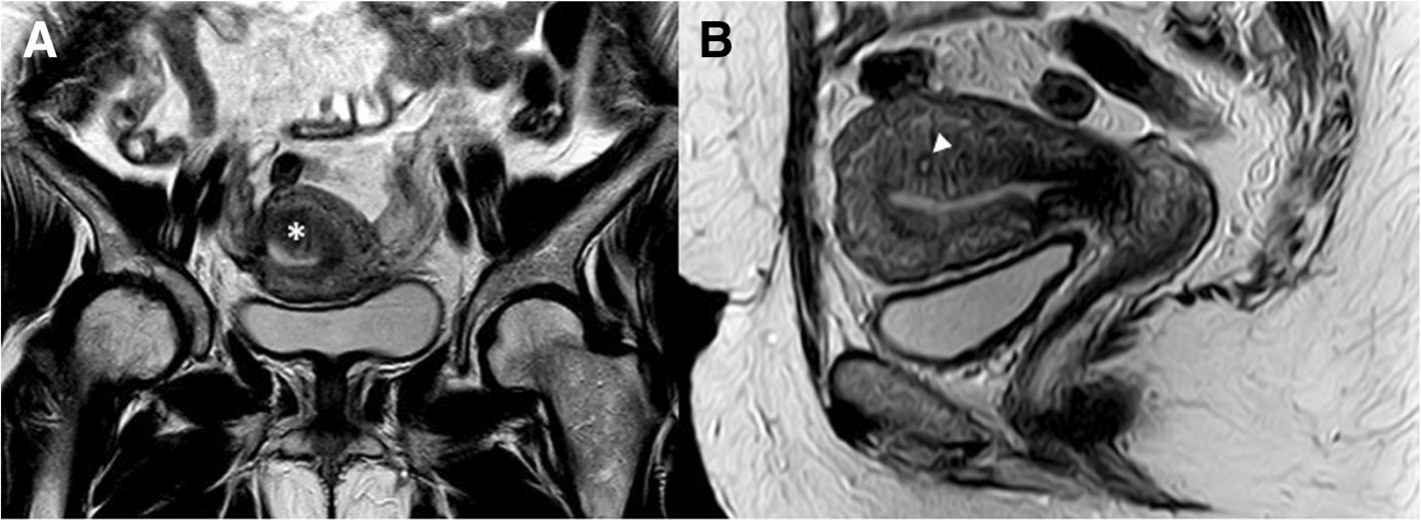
Coronal (a) and sagittal (b) T2W 1.5-T pelvic MRI images of a 42-year-old female with persistent pelvic pain following cesarean section show focal thickening of the posterior uterine wall transitional zone (asterisk) with tiny myometrial cyst (solid arrow head), suggesting focal adenomyosis
